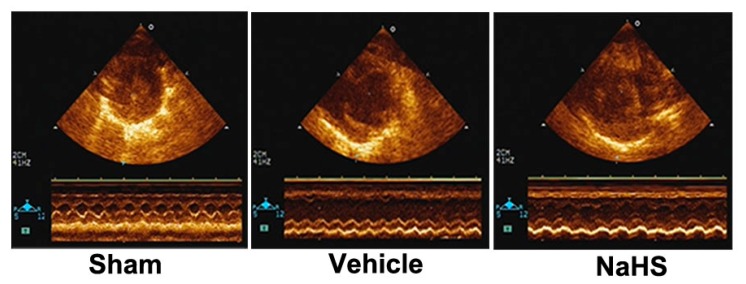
NaHS prevented cardiac dysfunction. Echocardiography assessment of cardiac function. Representative images of echocardiography. *n* = 7.
